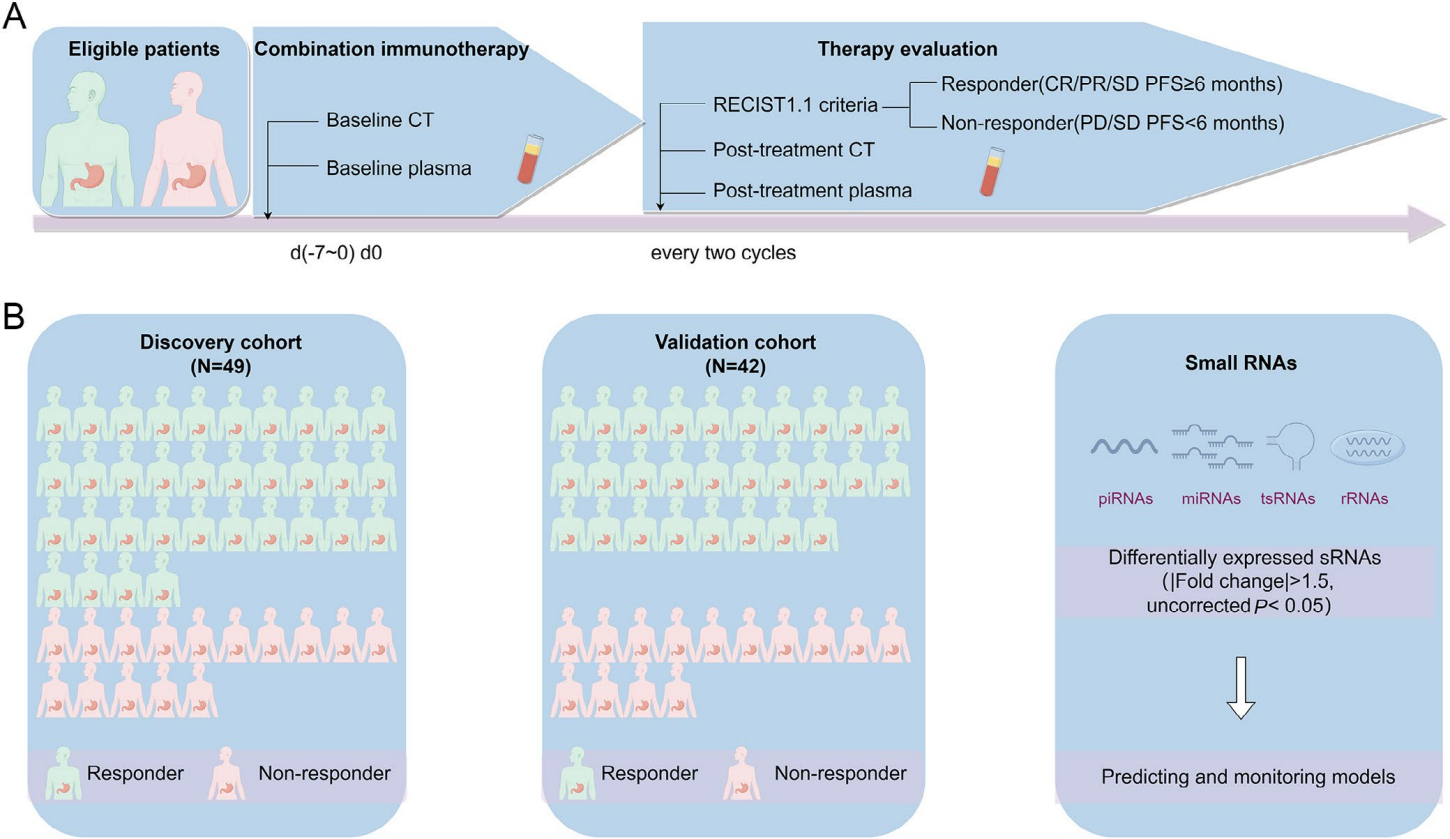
Study design. (A) Baseline plasma samples from patients with advanced gastric cancer were collected prior to the initiation of combination immunotherapy. Tumor status was evaluated by computed tomography (CT) every two cycles. Based on RECIST 1.1 criteria, patients were classified as responders (complete response (CR), partial response (PR), or stable disease (SD) with progression‐free survival (PFS) of ≥ 6 months) and non‐responders (SD with PFS ≤ 6 months or progressive disease (PD)). Post‐treatment plasma samples were collected at each evaluation point. (B) The discovery and validation cohorts included 49 and 42 patients, respectively, whose plasma samples underwent small RNA sequencing.
